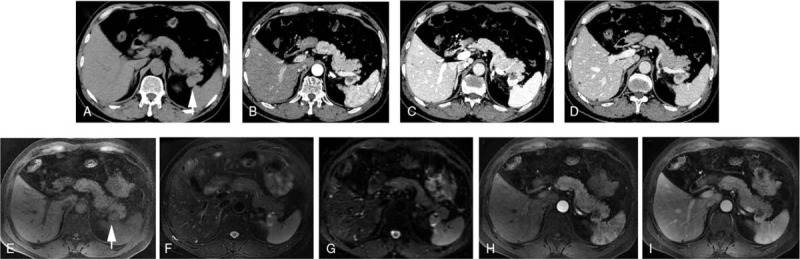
A well-defined cystic lesion (arrow) is revealed in the pancreatic tail. The Contrast-enhanced CT (A–D) and contrast-enhanced magnetic resonance imaging (E–I) demonstrate the enhanced pattern in all phases. (A) Precontrast CT; (B) arterial phase; (C) portal phase; (D) delayed phase; (E) T1-weighted imaging; (F) T2-weighted imaging; (G) diffusion-weighted imaging; (H) arterial phase; and (I) portal phase. CT = computed tomography
